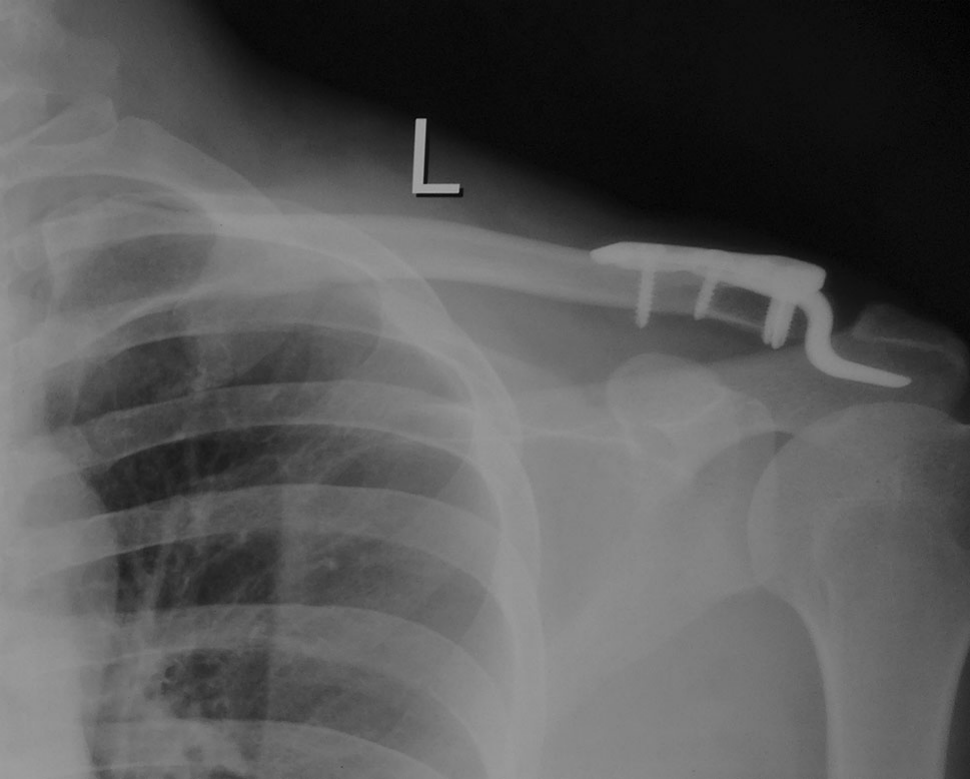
Post-operative radiograph at 12 weeks showing a congruent acromioclavicular joint and hook plate in situ
